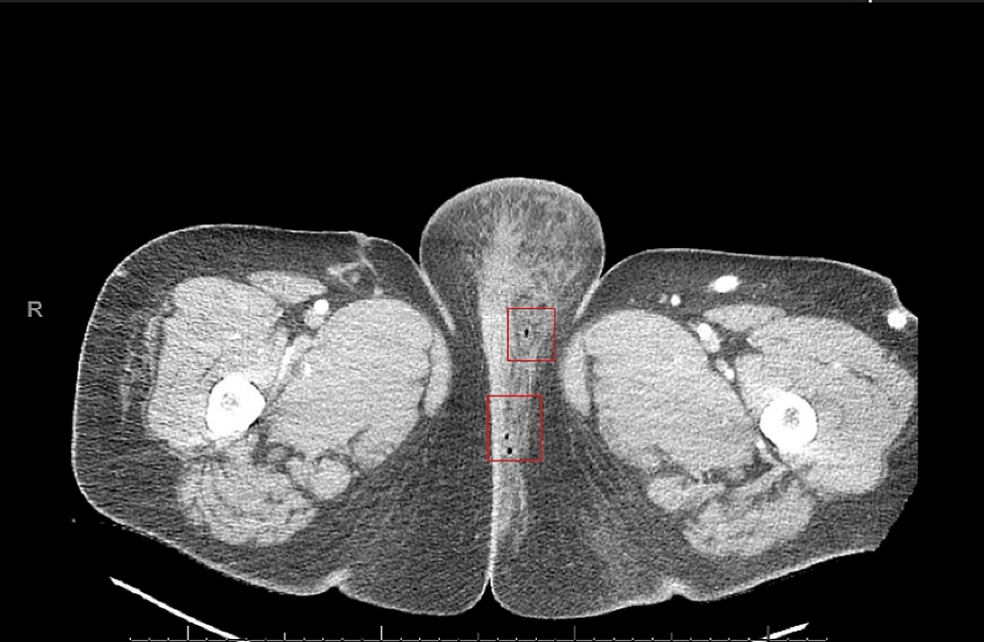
Contrast CT of the pelvis confirming disease progression Worsening left labial cellulitis with interval development of subcutaneous air (red boxes) concerning for an aggressive soft tissue infection
